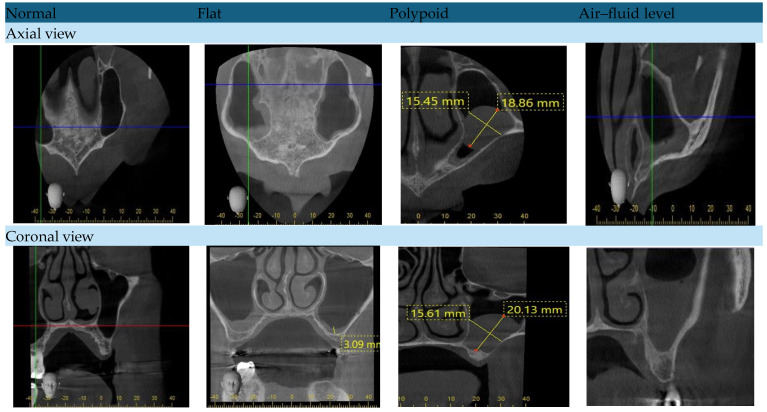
Examples of the varying mucosal thickening morphologies seen in axial and coronal views on CBCT.
